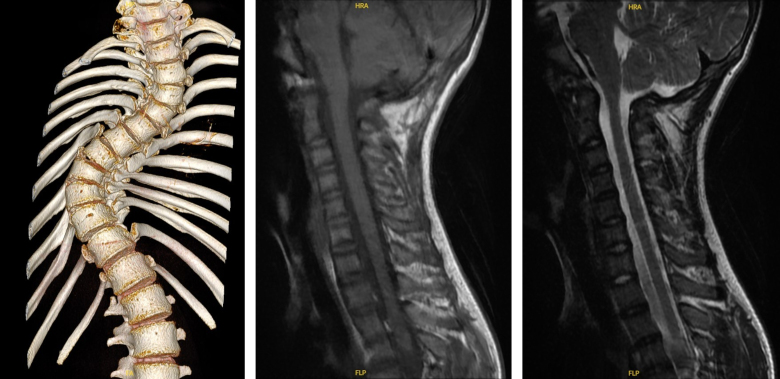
Three-dimensional, reconstructed computed tomography scan of the thoracic spine and cervical magnetic resonance imaging showing no obvious congenital vertebral malformations or cervical intraspinal abnormalities. FLP = foot–left–posterior, HRA = head–right–anterior.
